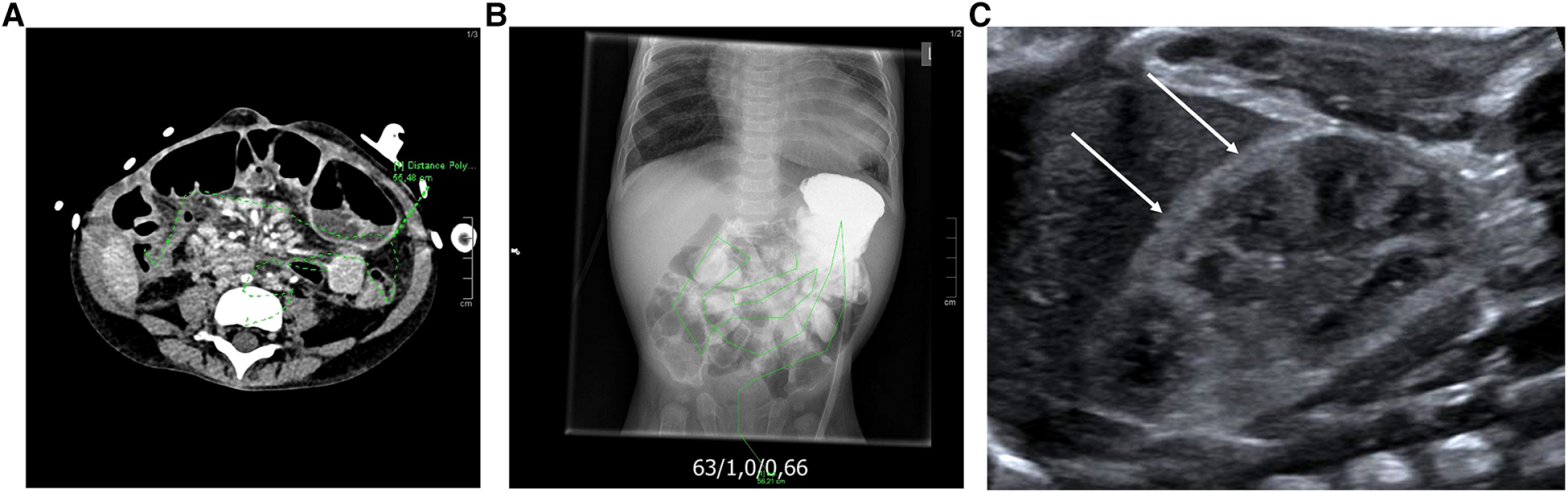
Representative determination of small bowel length in abdominal CT (A) and conventional x-ray (B) as well as ultrasonographic imaging (C) showing hyperechoic cortical halo (arrow) of the right kidney in patient 1 at the age of ca. 3 months, compatible with a beginning cortical nephrocalcinosis.
